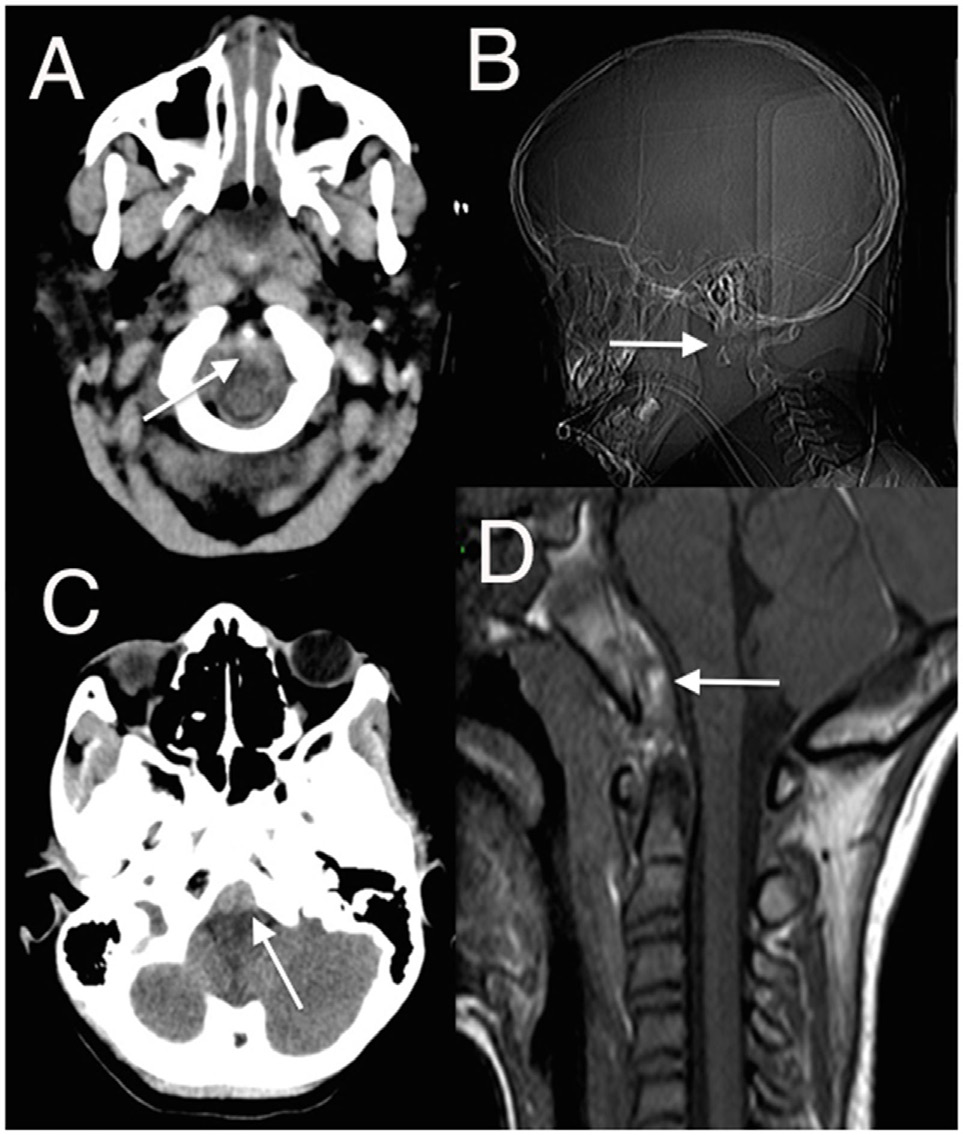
(A,C) Axial head computed tomography (CT) scan with low posterior fossa cuts revealing the retroclival hematomas anterior to the lower brainstem (arrowed); (B) CT surview showing atlanto-axial dislocation (arrowed); (D) sagittal T1 magnetic resonance imaging (MRI) showing retroclival hematoma (arrowed) better demonstrated on the subsequent MRI (13) (modified).
